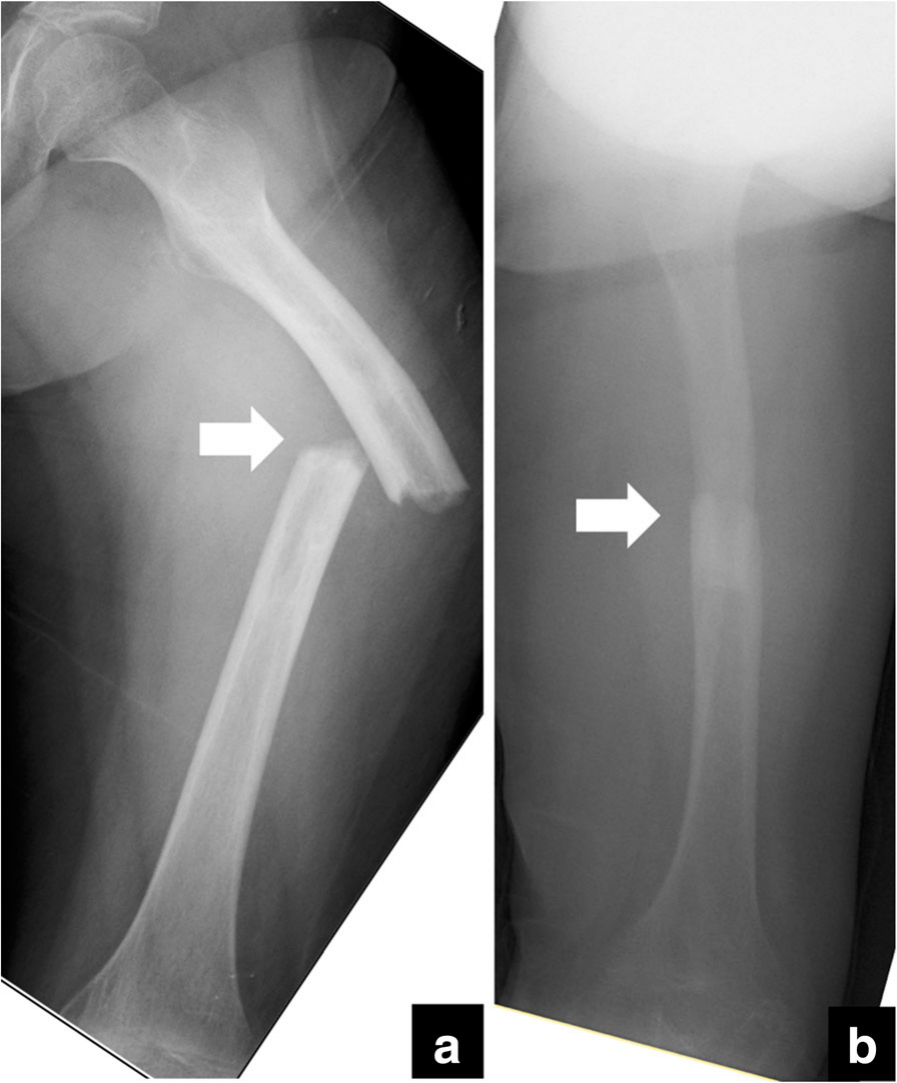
Radiographs of the left femur at the first refracture at a pin-site. (arrow) (a) the anteroposterior view, (b) the lateral view
